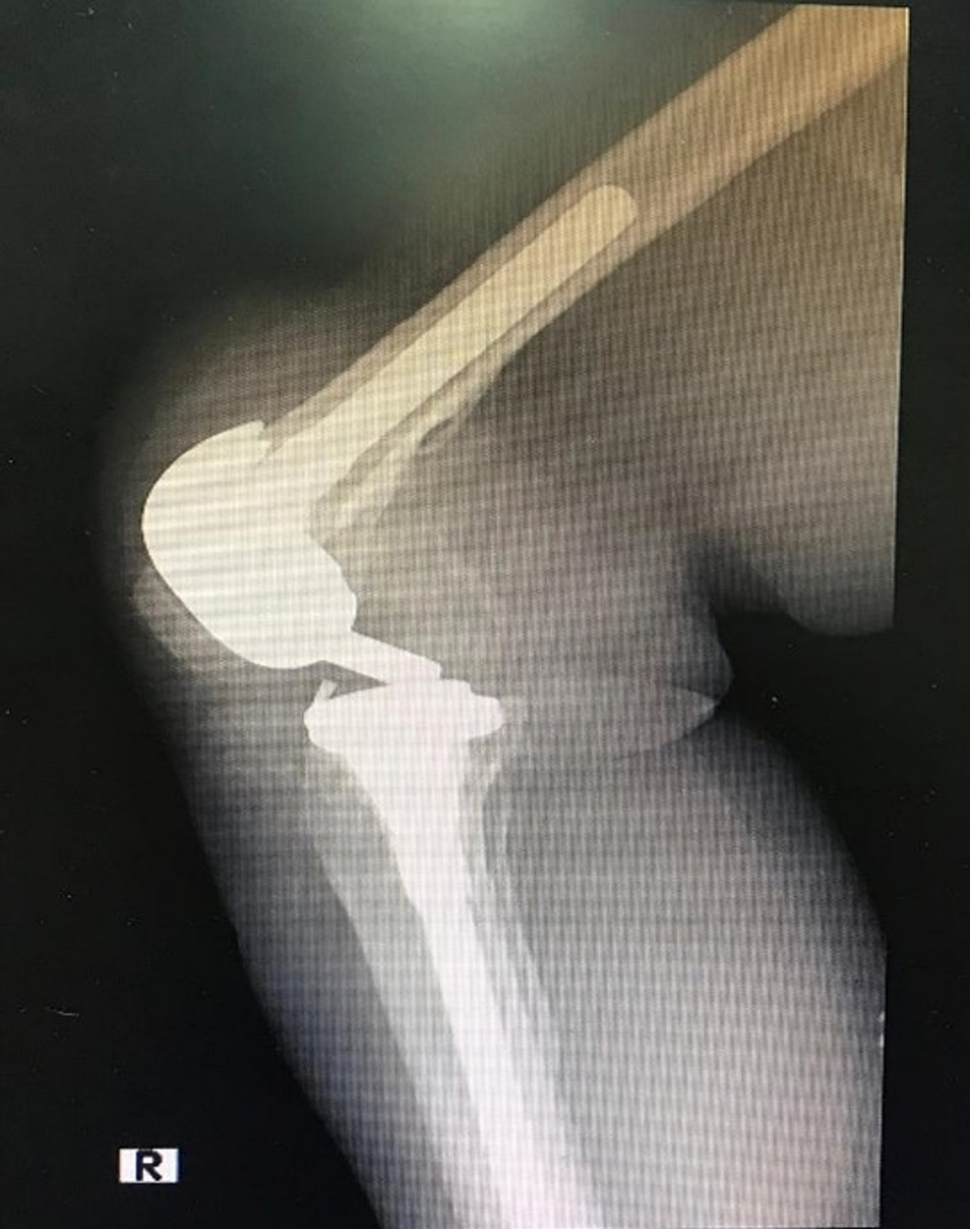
X-ray of the knee post total knee replacement: lateral view.
